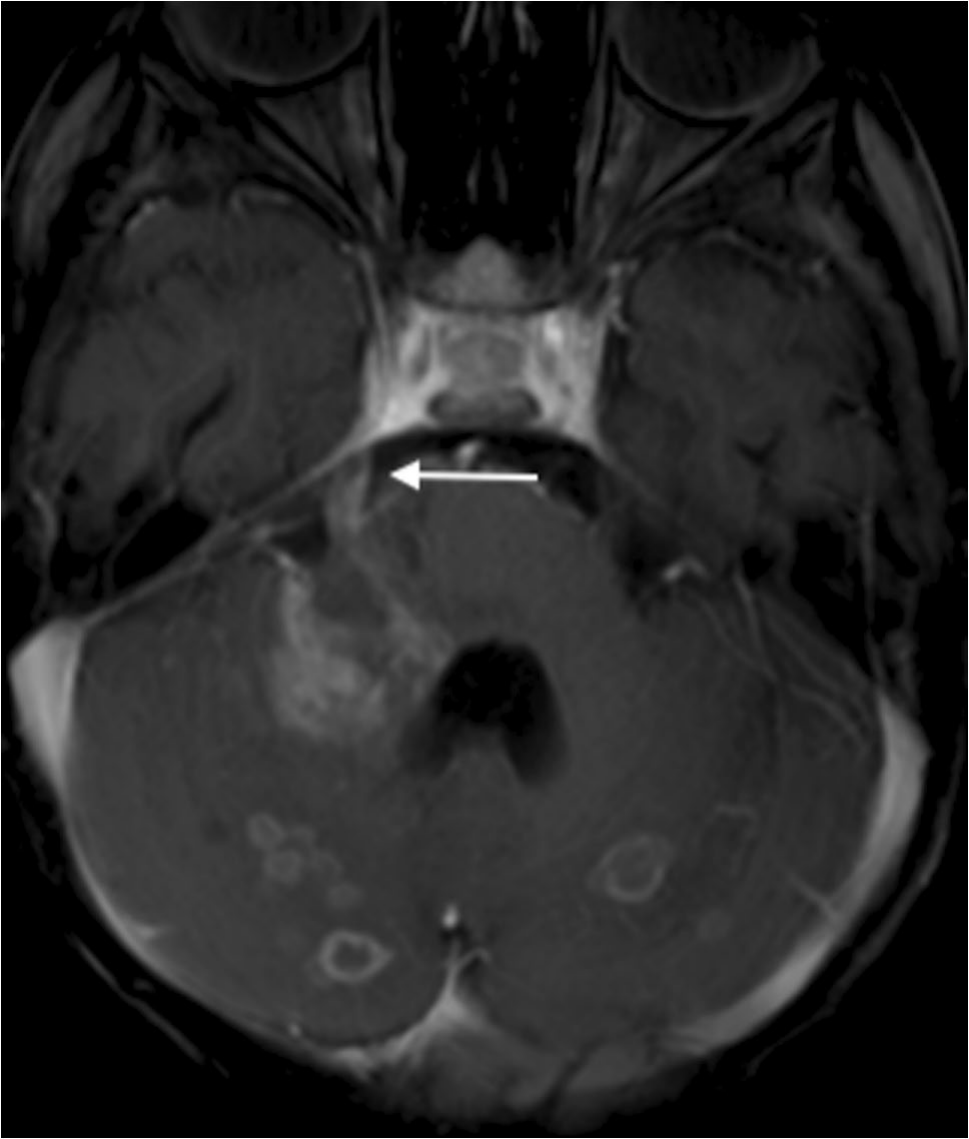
Axial T1 post-gadolinium magnetic resonance image in a 3-year-and-4-month old girl shows enhancement of the right trigeminal nerve (*arrow*). There is enhancement of the right cerebellar peduncle and there are multiple peripherally enhancing tuberculomas
